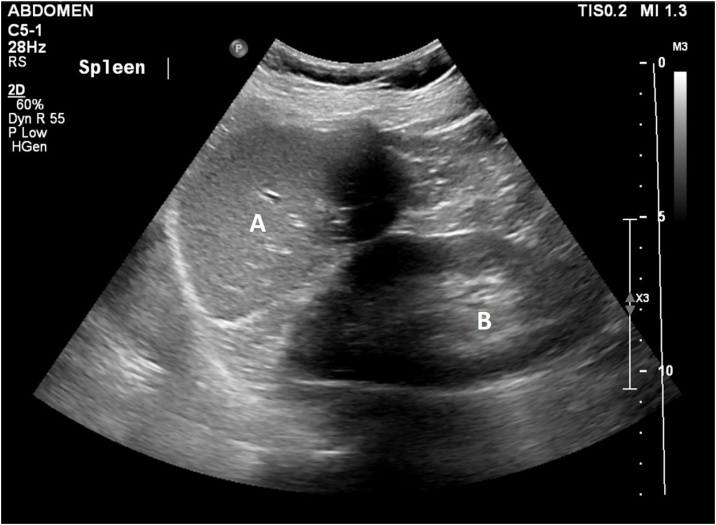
Abdominal ultrasound of left upper quadrant showing (A) spleen and (B) left kidney.
